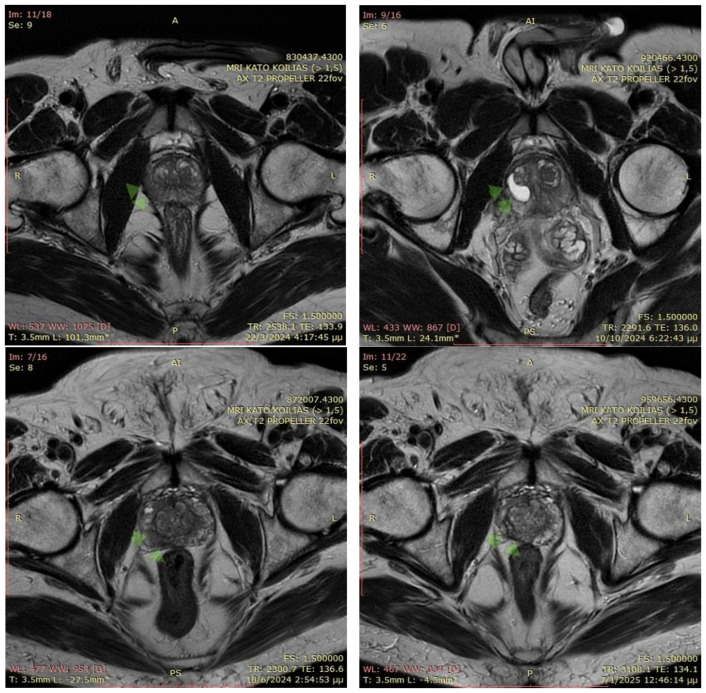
MRI of two patients pre-biopsy and after the EchoLaser therapy with fiducial markers procedure. The arrows in the figure indicate the locations of the lesions.
